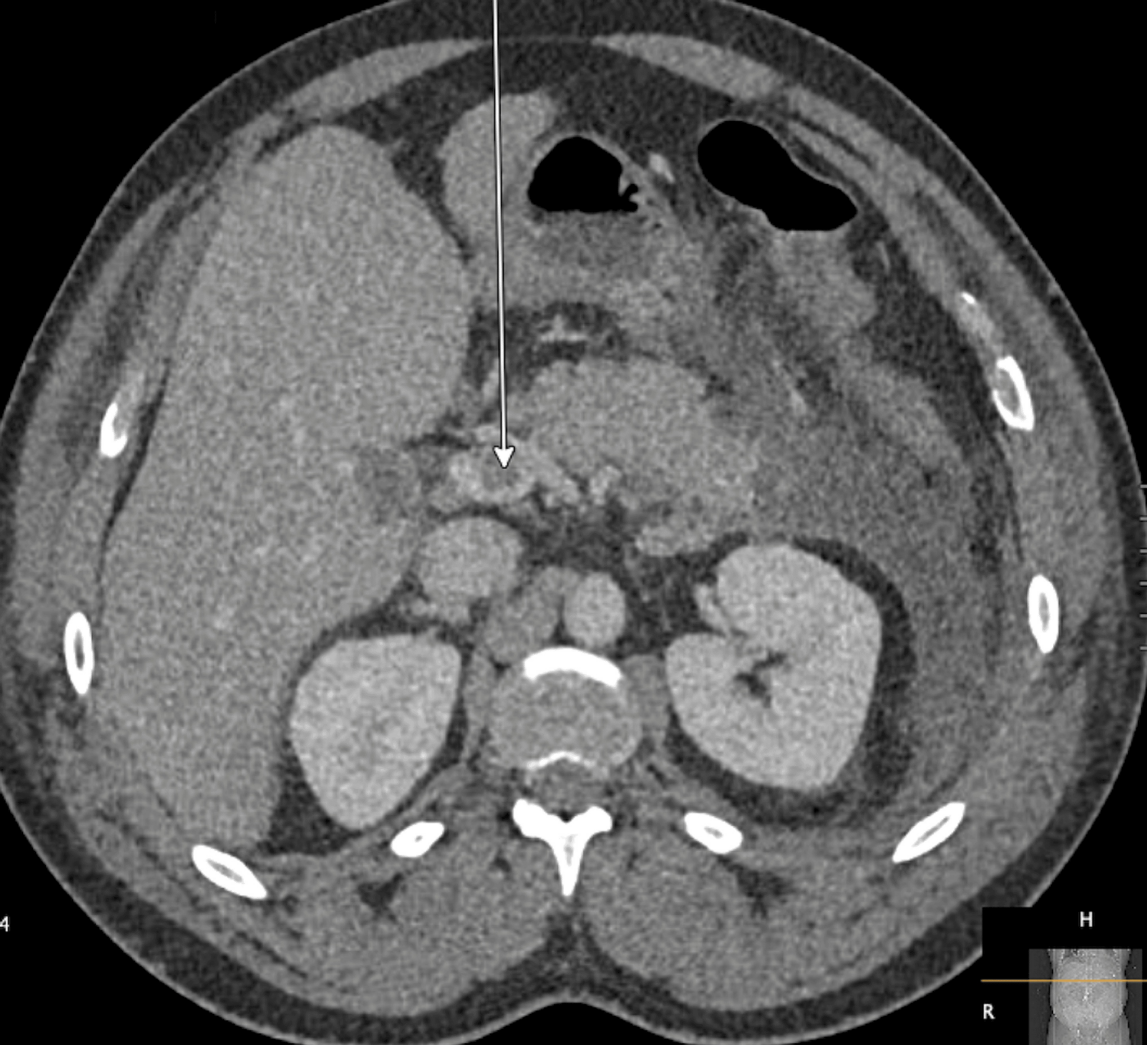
CT abdomen and pelvis with white arrow indicating the nonocclusive filling defect in the proximal portal vein, consistent with PVT CT: computed tomography; PVT: portal vein thrombosis
